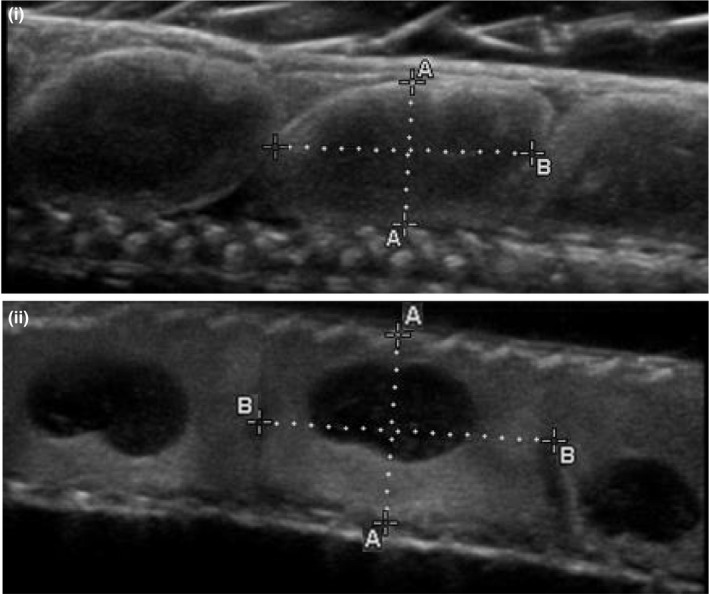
Ultrasound image of eggs within the same *Thamnophis elegans* female captured on (i) 16 June 2016, and (ii) again on 7 July 2016. Embryonic development in (ii) is more advanced than that shown in (i), and this image highlights the (approx. 92%) increase in volume, with eggs more tightly packed against the confines of the maternal abdomen and adjacent eggs
